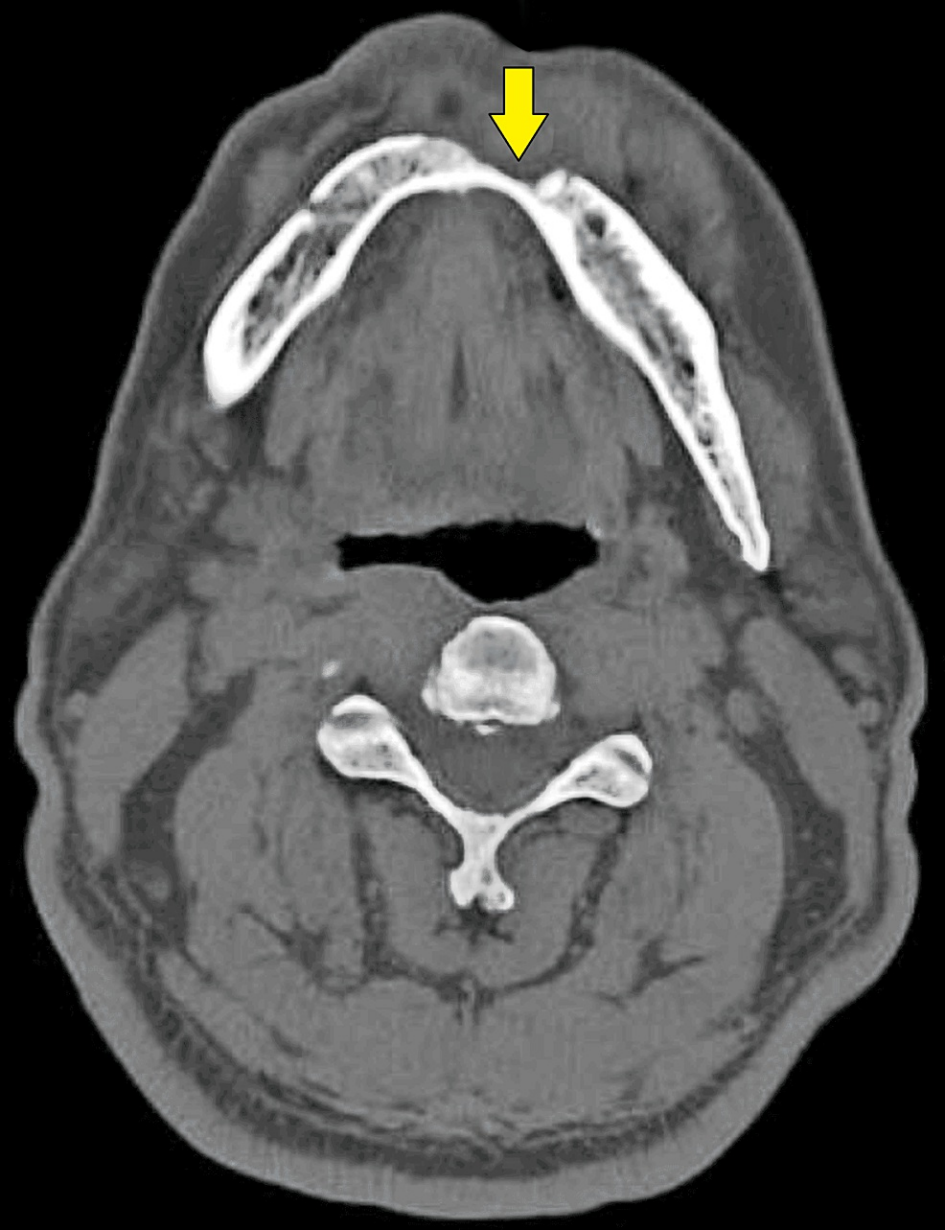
Evidence of bony erosion along with computed tomography scan (yellow arrow)
